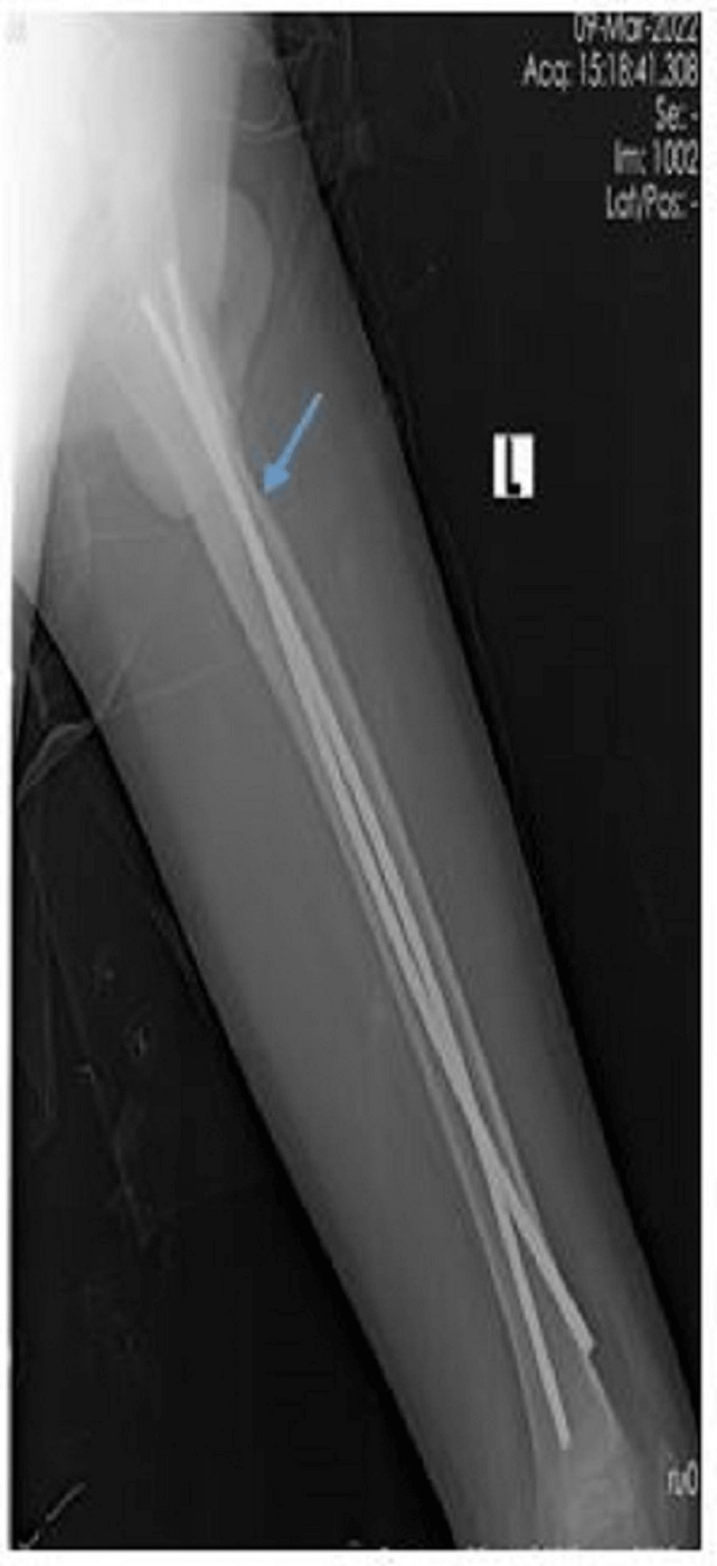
Lateral radiograph of a nine-year-old male who underwent TENS fixation for subtrochanteric fracture (blue arrow) with fracture union TENS: titanium elastic nailing system
